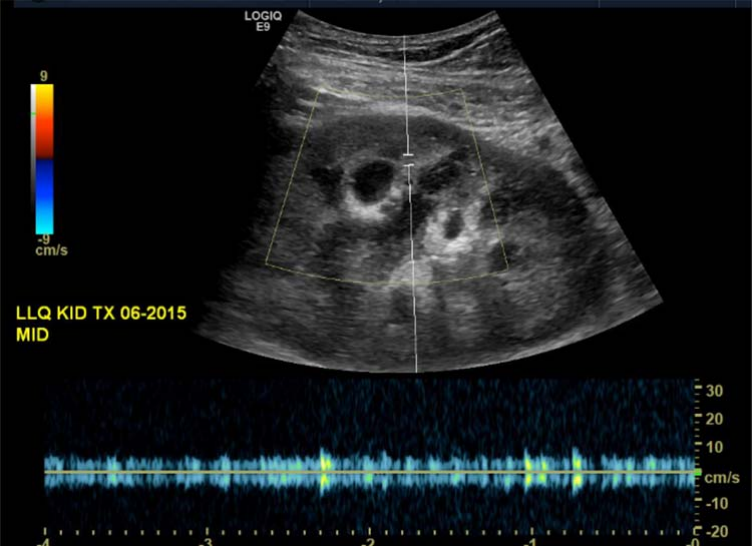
Ultrasound of the transplanted kidney showing no blood flow within the main renal artery or vein.
